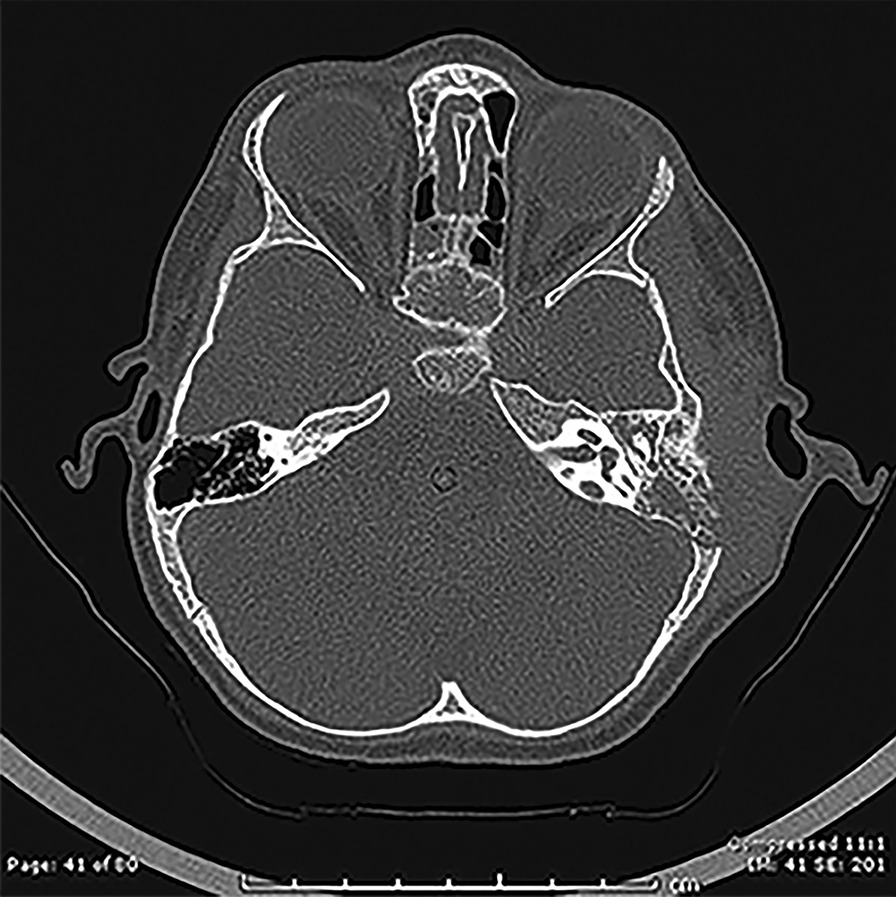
CT scan of the head: showed evidence of left side mastoiditis, opacification of the middle ear cavity and the mastoid air cell, with destruction of the mastoid septae and lateral wall and postauricular swelling
